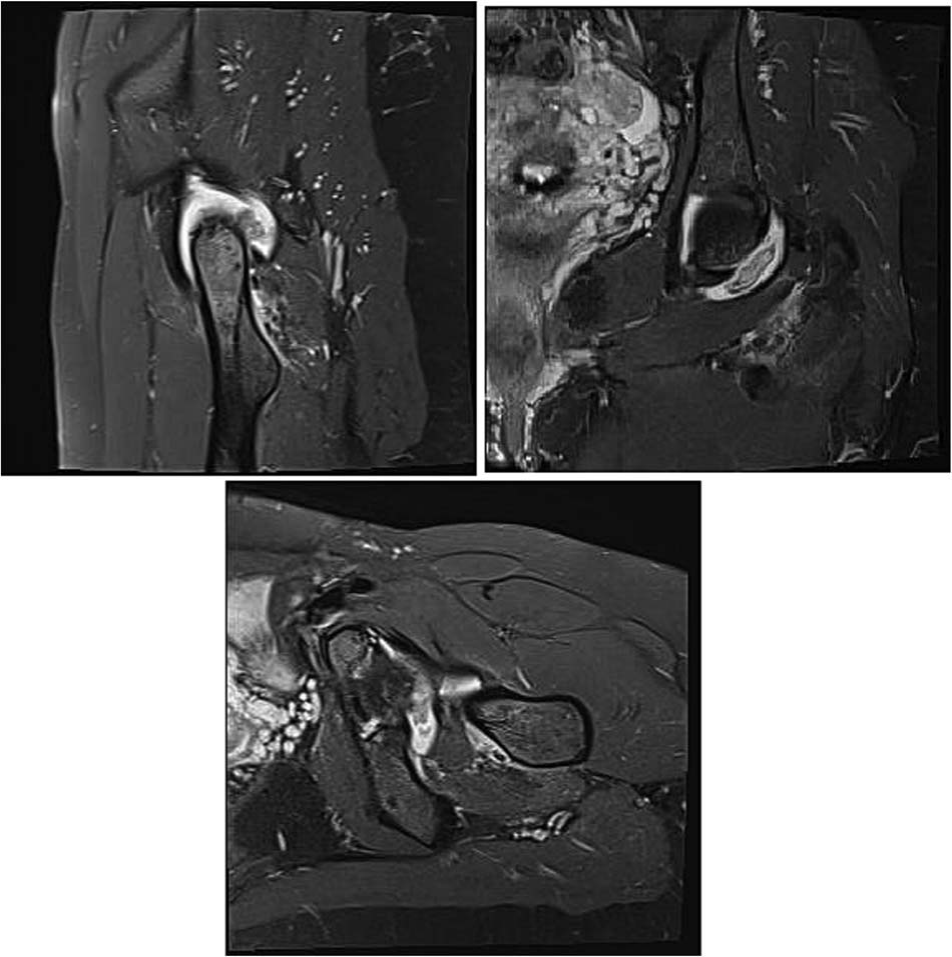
MRI is observed in axial, sagittal, and coronal sections, showing the presence of an intra-articular lesion and abundant synovitis.
